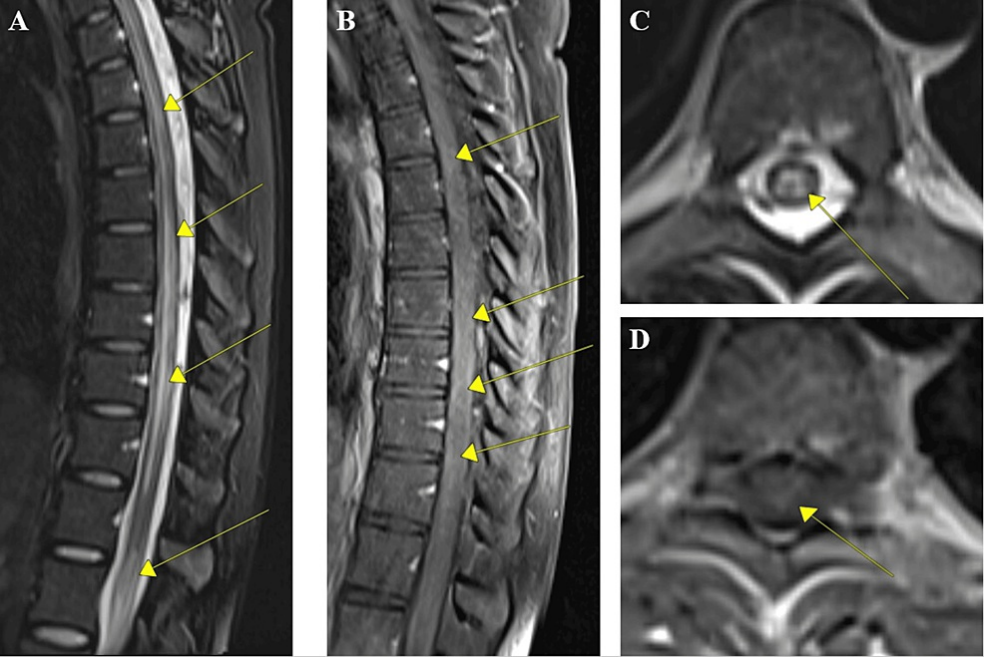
MRI of the thoracic spine with and without contrast. Diffuse abnormal T2 hyperintensity through the spinal cord from the lower cervical spine to T11 and in the distal conus medullaris. A-B) MRI of thoracic spine sagittal shows several longitudinally extensive lesions with mild pathological contrast enhancement. C-D) Axial MRI of thoracic spine imaging at T8 with hyperintensity and mild contrast enhancement.
